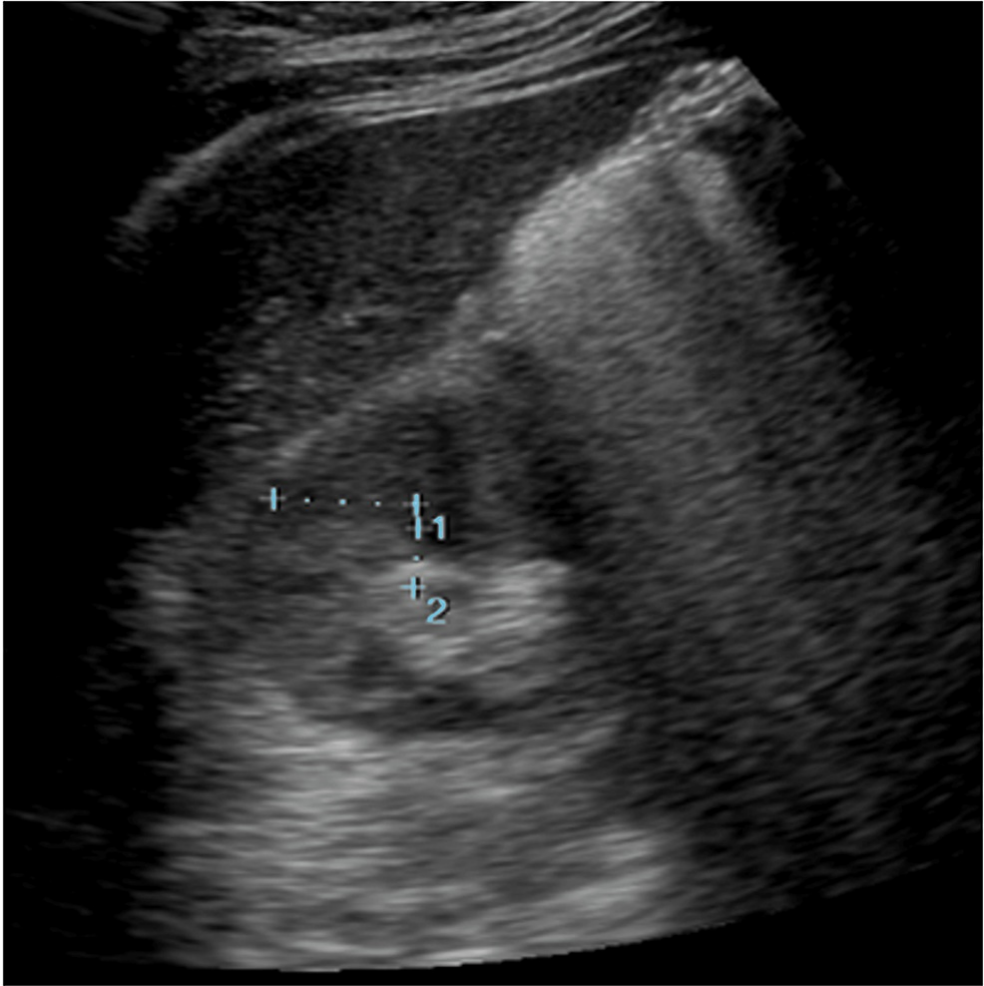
Abdominal ultrasonography showing a shrinking, slightly hypo-absorptive zone in the right kidney (15 × 7 mm) This was four weeks after the first ultrasonography (shown in Figure 1).
